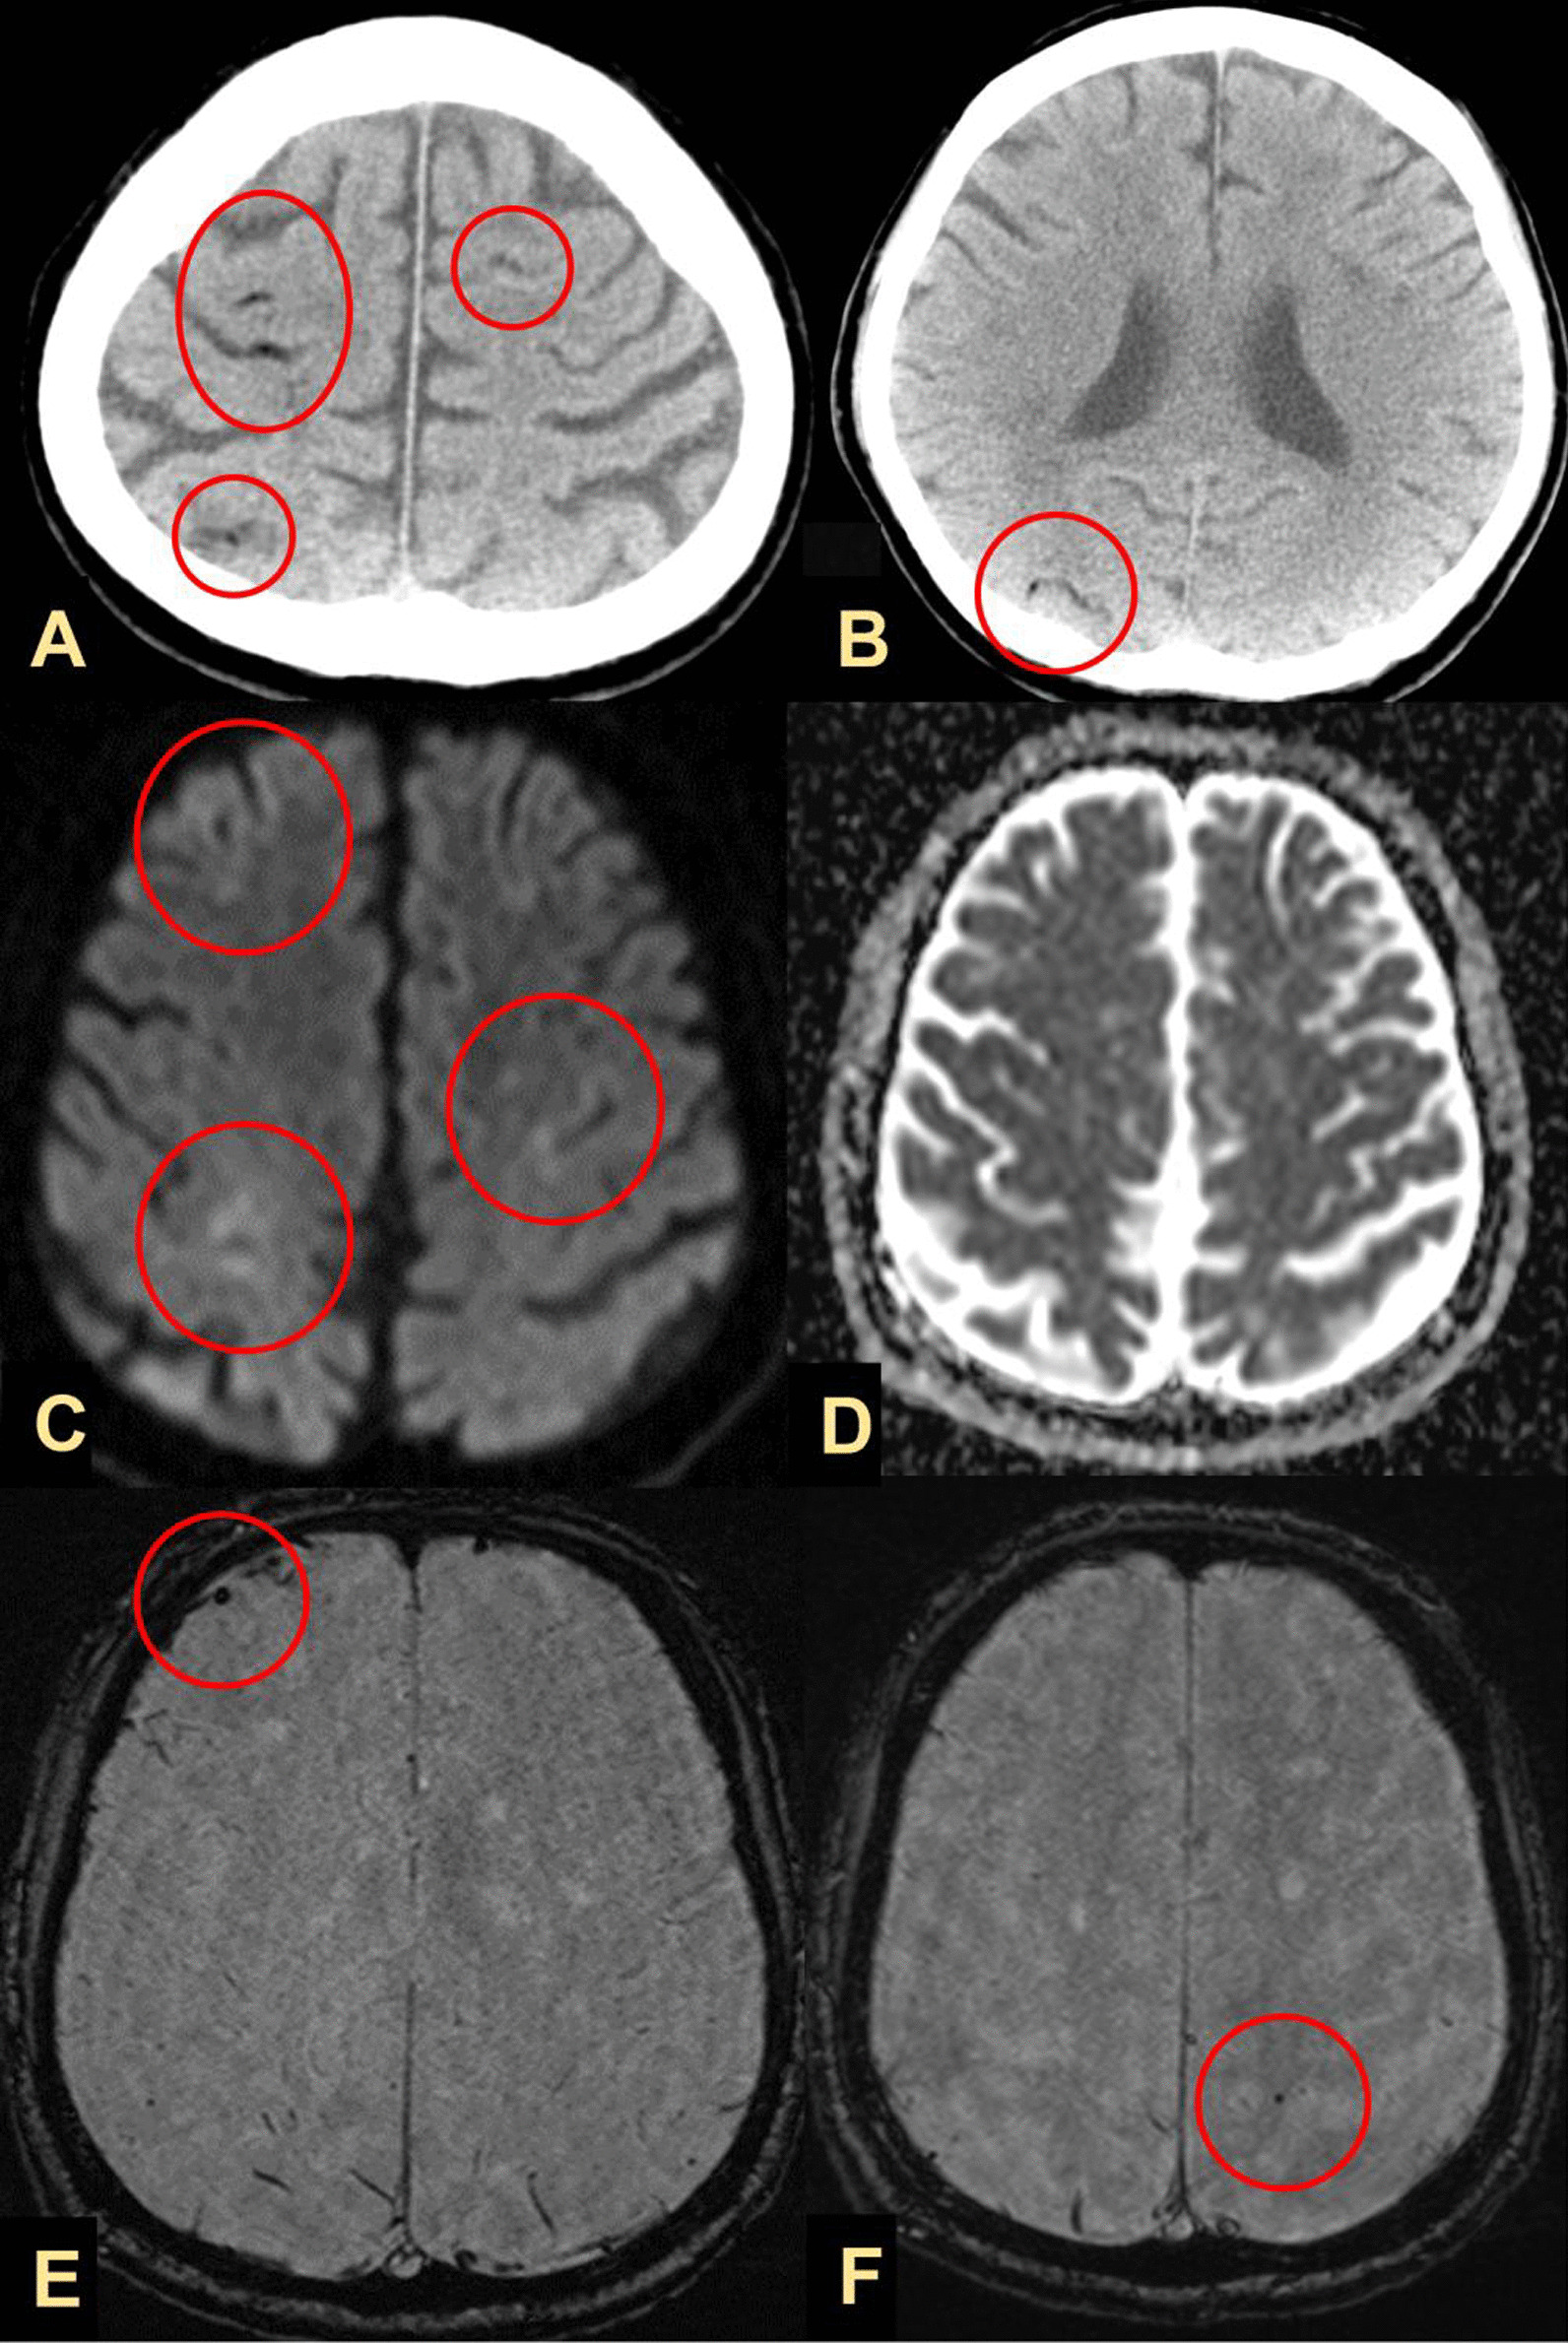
Head computed tomography (CT) image taken on day 1 showing multiple air-like densities (circle) in the right cortex and the subcortex bilaterally (A, B). Window level/window width: (40/80). Head magnetic resonance imaging (MRI) showing faint hyperintensity (circle) along the right parietal cortex and subcortex and the left frontal cortex (C) on diffusion-weighted images, with an apparent diffusion coefficient map (D) and a small dot-like low signal (circle) in the right frontal cortex (E) and the left parietal subcortex (F) on susceptibility-weighted imaging. (TR/TE): C, D: (4000/95), E, F: (49/40)
